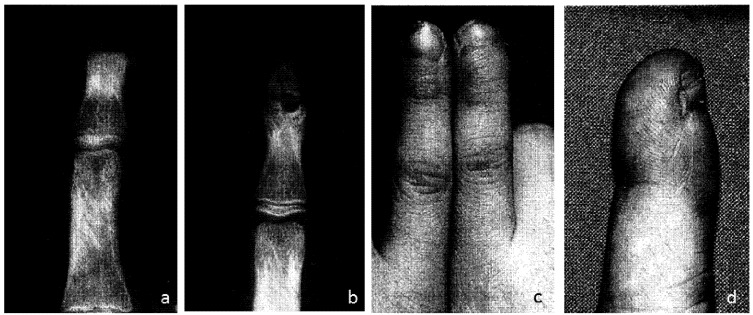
X-rays of patient at admission (a) and six months after the injury (b) showing regeneration of the distal phalanx. Photographs of the injured finger six months after the injury with left index finger for comparison (c) and close up (d) (Adapted from [16]).
